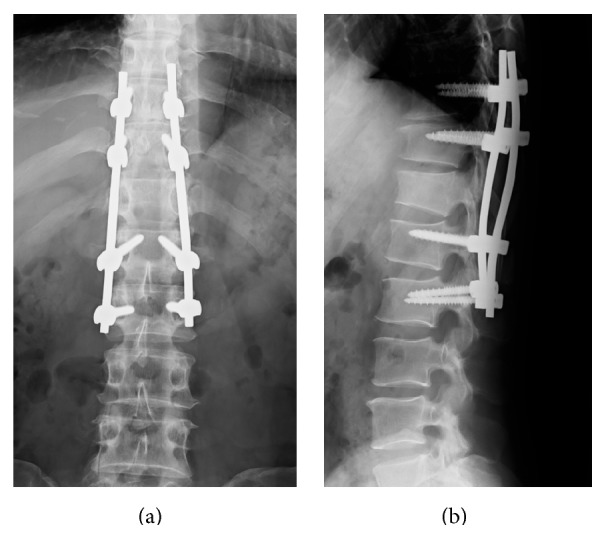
Postoperative radiographs (at final follow-up and 6 months after revision surgery). (a) AP view, (b) lateral view. X-ray at final follow-up revealed a nearly healed T12 fracture.
